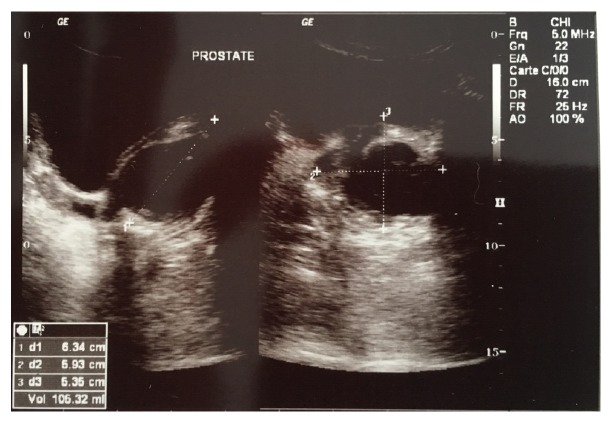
Abdominal ultrasound showing hypoechoic collection inside prostatic parenchyma.
